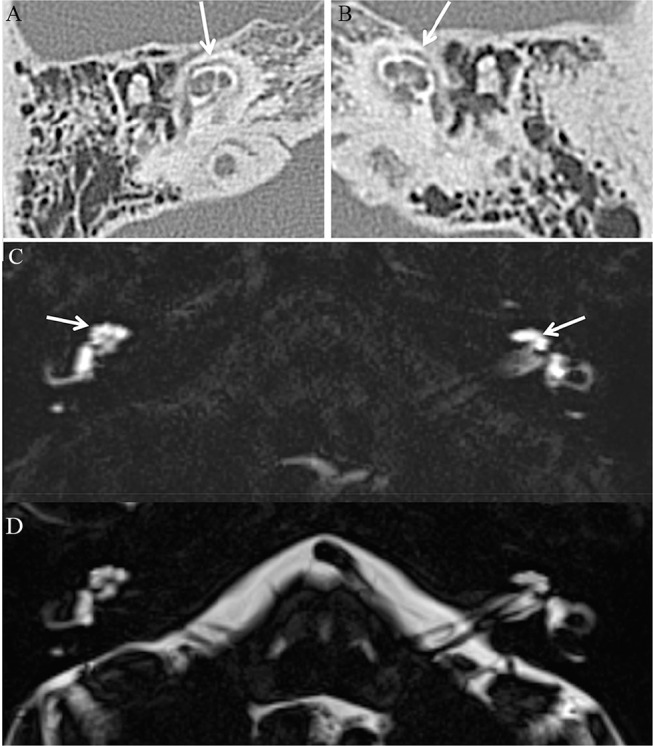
A 53-year-old man with bilateral retrofenestral otosclerosis. (A, B) On computed tomography images, otospongiotic plaques around the cochleae are visualized as low density bands (arrows). (C) Heavily T_2_-weighted three-dimensional fluid-attenuated inversion recovery (3D-FLAIR) image obtained 4 hours after intravenous administration of a single dose of gadolinium-based contrast agent (IV-SD-GBCA). Remarkably high signal is seen bilaterally in the cochleae (arrows). In this particular subject the signal intensity ratio (SIR) was 47 in the right cochlea and 40 in the left cochlea. (D) Magnetic resonance cisternography (MRC) at the slice level corresponding to that in (C).
